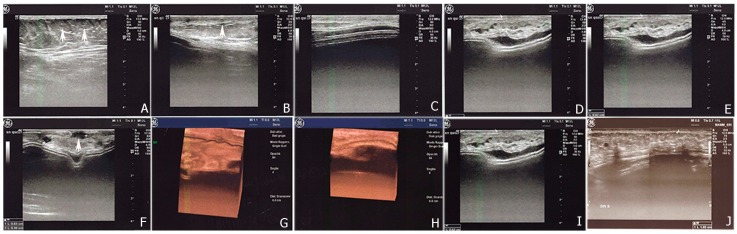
Ultrasonography figures showed a good visualization of the implanted adipose parenchyma showing internal echotexture inhomogeneous (A–C) and with the presence of small areolas hypo-anechoic with diameter max up to about 0.6 cm (F). 3D-4D scans show no structural alterations parenchyma (G,H). The prosthesis is discreetly located with irregular profiles and wavy characters without contracture, with the exception of some small areas characterized by thickening and splitting of the margin (D–F). The thickness of adipose tissue implanted is about 0.52 cm after lipofilling I and about 1.0 cm after the end of treatment (I,J).
